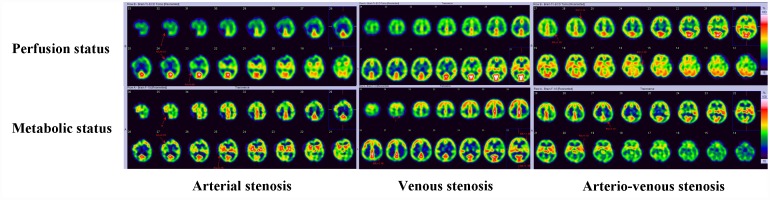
Perfusion and metabolic status of CAS, CVS and CAVS on SPECT. CAS and CAVS always has focal or unilateral reduced perfusion with elevated or reduced metabolic status. CVS displays with bilateral symmetrical perfusion reduction and metabolic status maintaining normal or elevation frequently.
